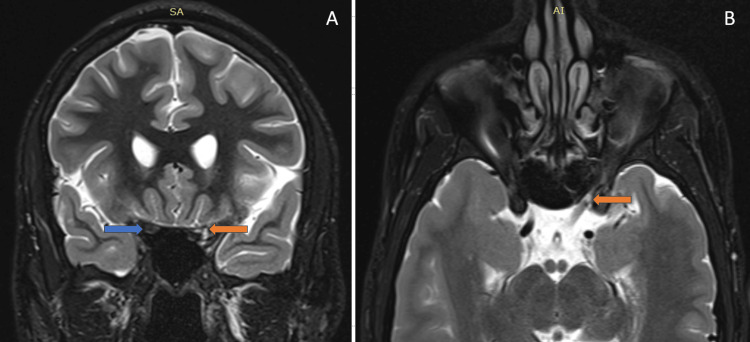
(A) Coronal MRI short tau inversion recovery (STIR) image shows fluid signal intensity in the intracanalicular segment of the left optic nerve suggestive of avulsion/tear of the left optic nerve (orange arrow) compared to the normal signal intensity of the right optic nerve in the optic nerve (blue arrow). (B) Axial MRI STIR image shows fluid signal intensity in the intracanalicular segment of the left optic nerve suggestive of avulsion/tear of the left optic nerve (orange arrow).
